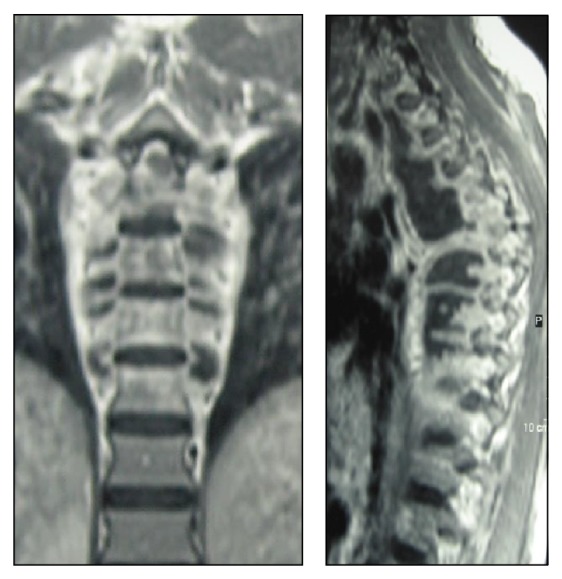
MRI with gadolinium showing a paravertebral abscess.
